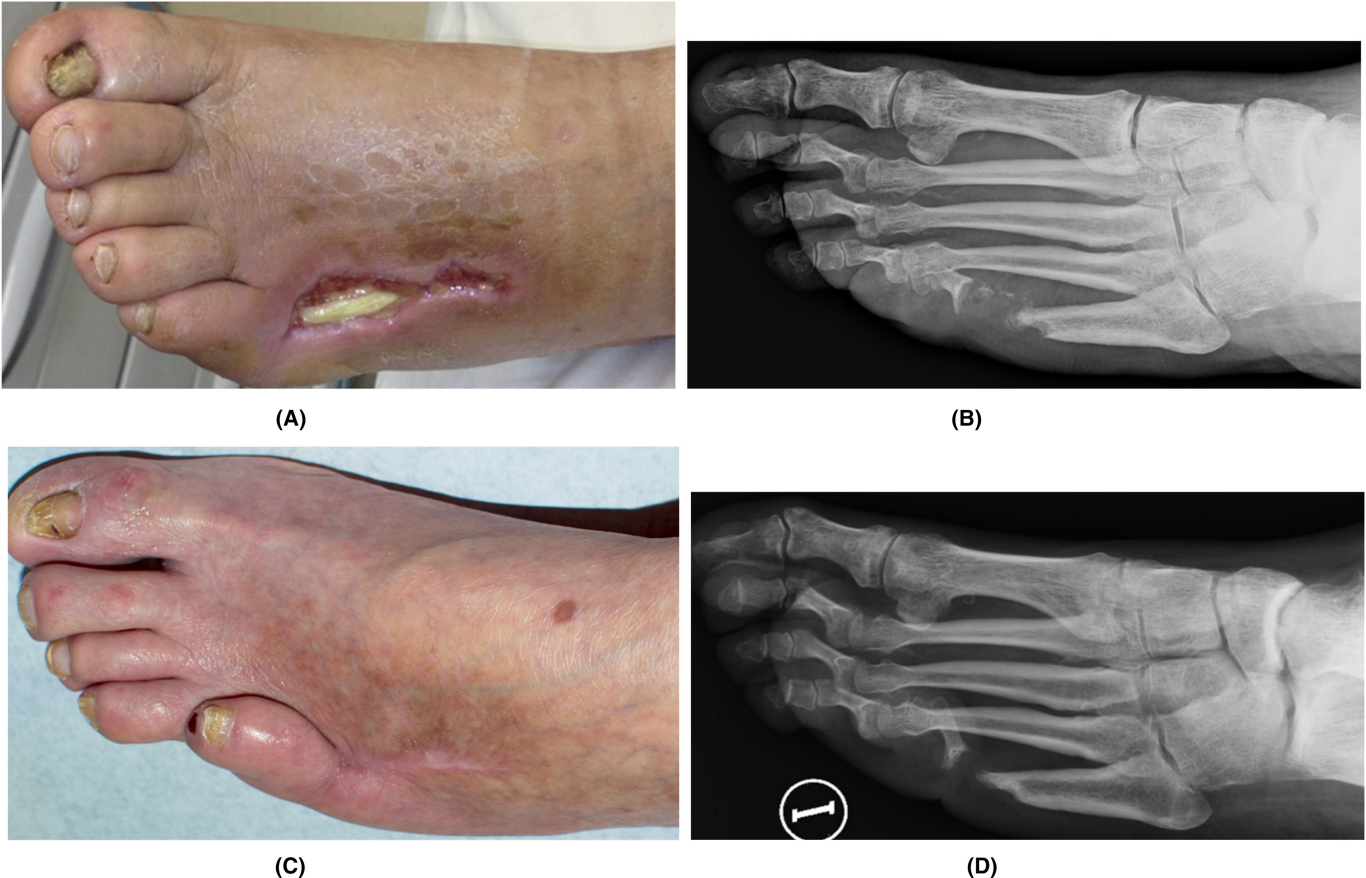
(A) A DFU with tendon exposure, osteomyelitis and fistula through the entire foot. (B) Radiograph at the start of the NGR‐T showing clear signs of osteomyelitis. The wound was completely closed after 7 weeks. (C) Control picture 5 years after wound closure without any recurrence of the ulcer, and with (D) the radiograph indicating a resolution of the osteomyelitis.
